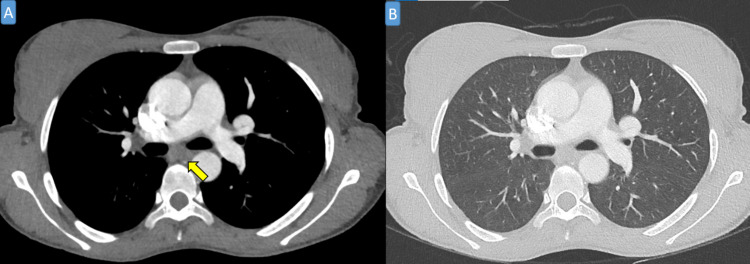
Axial computed tomography thorax with contrast in soft tissue window (A) and lung window (B) that shows no evidence of mediastinal lymphadenopathy (yellow arrow).
